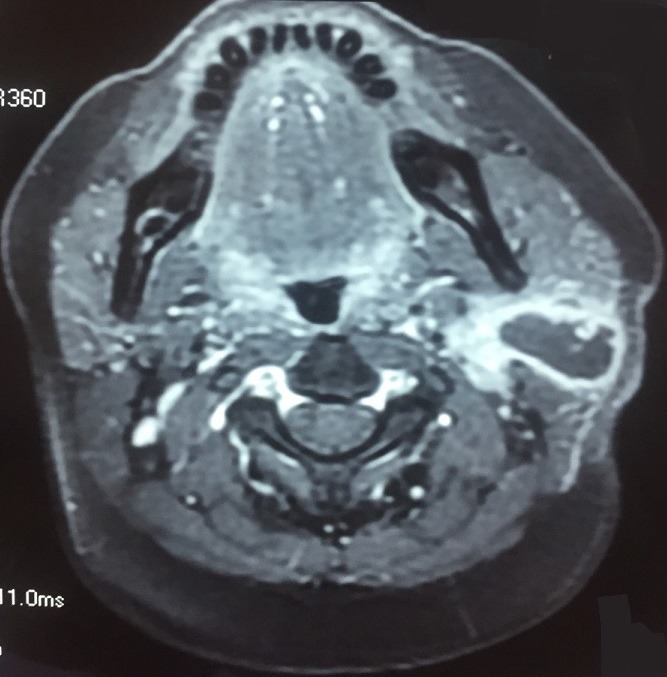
T1 axial section MRI after gadolinium injection
